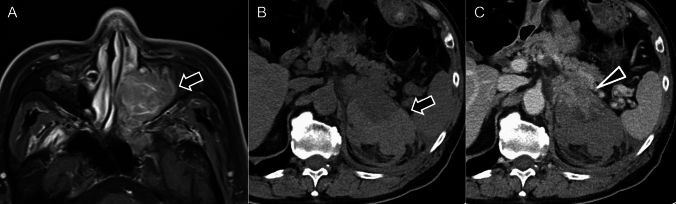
Imaging of adrenal metastasis. Patient with angiosarcoma of the maxillary sinus. A Magnetic resonance imaging of the maxillary sinus showing a mass occupying the left-maxillary sinus with heterogeneous enhancement on gadolinium-enhanced T1-weighted imaging (arrow). B Unenhanced CT showing a high-density area dorsally to the left-adrenal mass, suggesting retroperitoneal hemorrhage (arrow). C Enhanced computed tomography showing irregular tumor enhancement in the left-adrenal mass (arrow), consistent with metastasis. Adrenal metastasis can occasionally be complicated by hemorrhage
